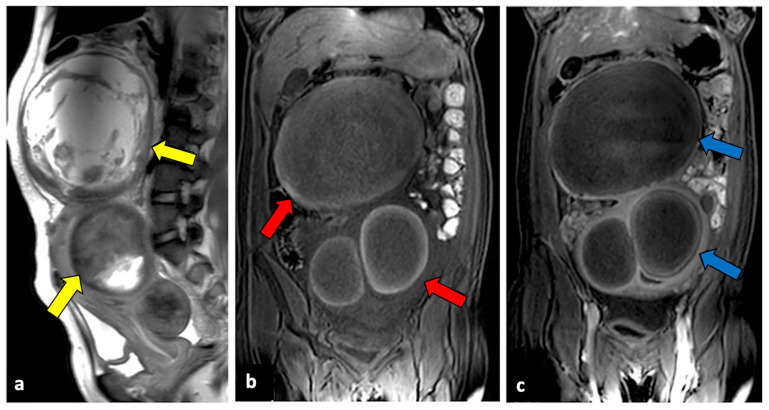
Red degenerated leiomyoma in a thirty-one-year-old woman with abdominal pain and vaginal bleeding after a miscarriage. T2-weighted imaging (a) showed an enlarged uterus with degenerated intramural and pedunculated leiomyomas with inhomogeneous intensity (yellow arrow). On T1-weighted sequence (b), a peripheral hyperintense rim surrounding the lesion’s central area of lower signal intensity (red arrows), typical of red degeneration of leiomyomas, is observed. No intralesional enhancement was seen after intravenous contrast injection ((c), blue arrows).
